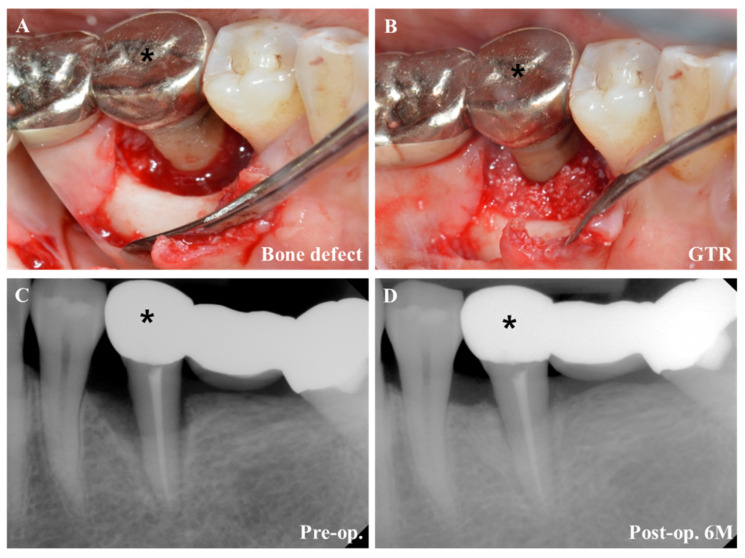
GTR. (A) Severe alveolar bone defect around tooth (*) is observed. (B) After cleaning the defect, bone grafting was performed. (C) Preoperative condition observed in radiograph. (D) Postoperative periodontal tissue regeneration was observed in radiograph.
